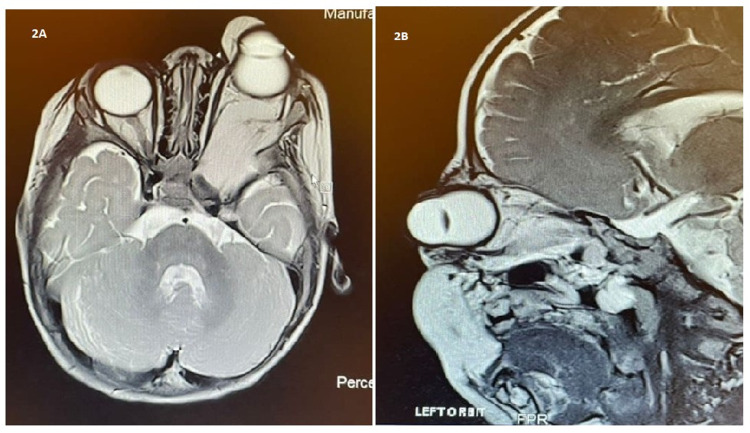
(A and B) Axial and sagittal MRI views showing left eye corneal dermoid and left-sided retrobulbar mass extending to the cavernous sinus
